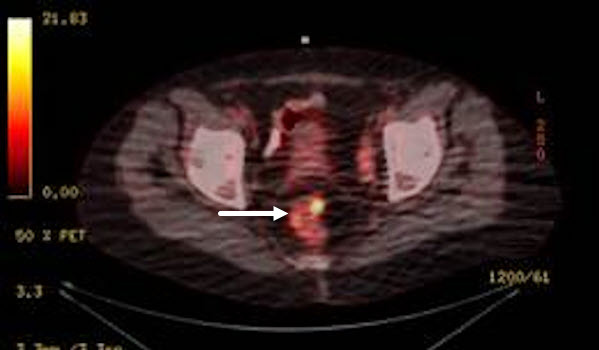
A PET-CT Scan Showing a Rectal Mass.
